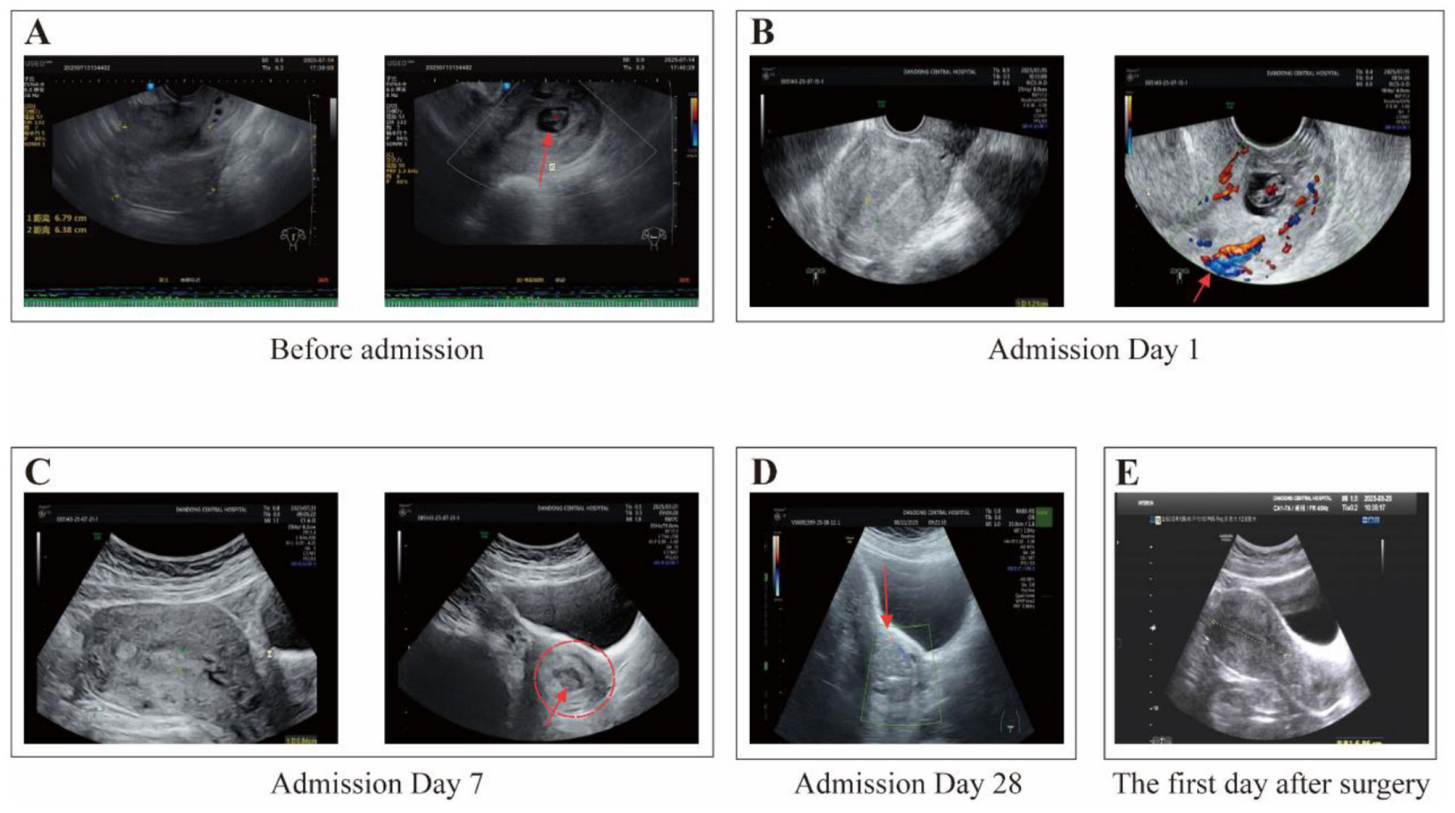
Serial TVUS of a cervical ectopic pregnancy managed with MTX plus adjunct TCM, followed by suction curettage. (A) Baseline (pre-admission): Grayscale TVUS shows a gestational sac implanted within the endocervical canal (→). (B) Hospital day 1: color Doppler confirms cervical implantation and reveals marked peritrophoblastic vascularity at the implantation site (→). (C) Hospital day 7 (after first MTX dose): Grayscale TVUS shows a non-viable pregnancy (○); the embryonic pole (→) exhibits no fetal cardiac activity, consistent with embryonic demise. (D) Hospital day 28 (after combined medical therapy, preoperation): Grayscale and color Doppler imaging reveal a heterogeneous echogenic mass within the cervical canal, measuring 4.0 × 3.3 × 3.0 cm by calipers. The previously prominent peritrophoblastic flow has regressed to scant focal signals (→), indicating substantial devascularization. (E) Postoperative day 5: follow-up grayscale TVUS confirms complete resolution of the cervical lesion with restoration of normal cervical and uterine anatomy. Interpretation: The series documents progressive devascularization and degeneration of trophoblastic tissue under medical therapy, facilitating safe definitive surgical evacuation. TVUS, transvaginal ultrasonography; MTX, methotrexate; TCM, traditional Chinese medicine.
